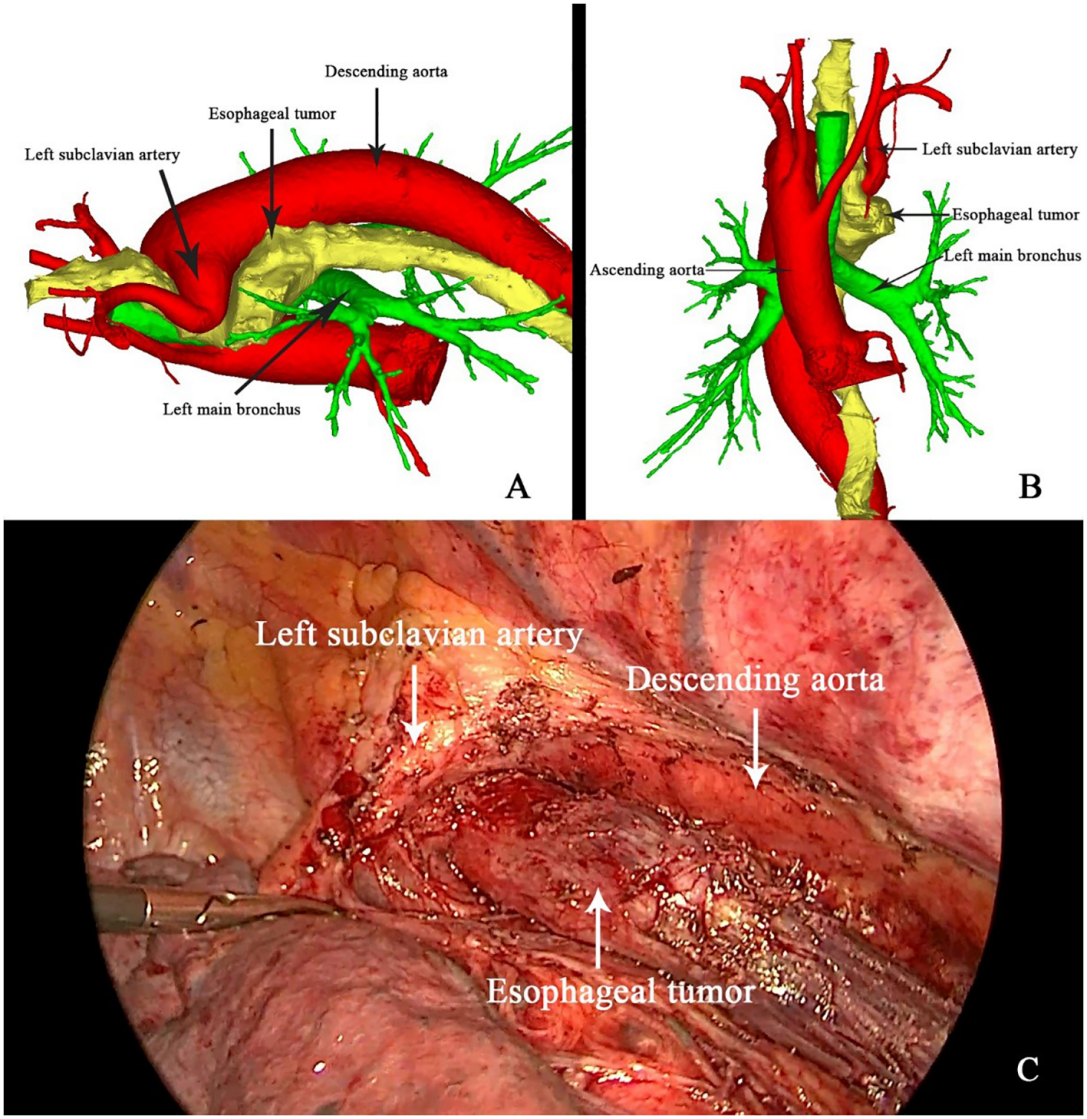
(A) Image of esophageal tumor under endoscopic ultrasound. (B, C) Comparison of esophageal tumor on CT images before and after neoadjuvant therapy.
